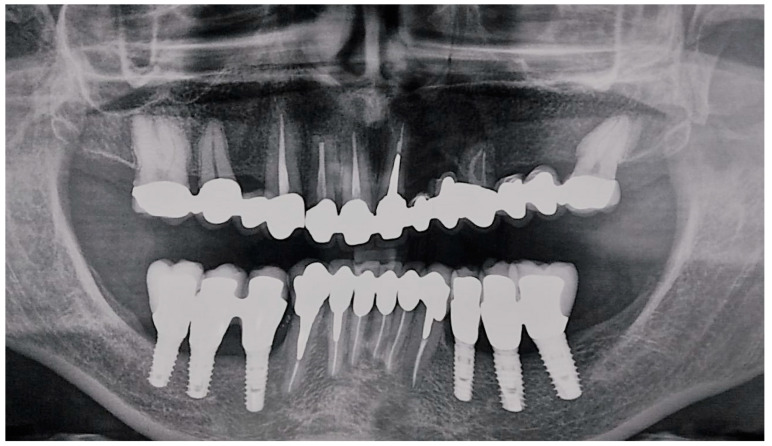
Twelve month follow-up: Orthopantomography.
